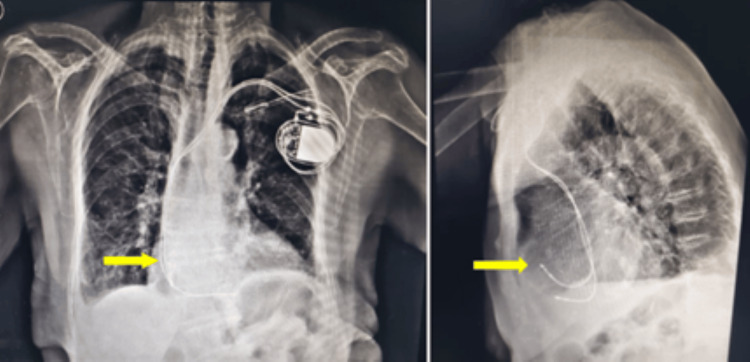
Posteroanterior and Lateral Chest Radiographs Chest radiographs (posteroanterior and lateral views) showing the pacemaker generator in the left subclavicular position with leads correctly positioned in the right heart chambers - the atrial lead anchored to the anterolateral wall of the right atrium and the ventricular lead directed toward the septal or apical region of the right ventricle. The cardiac silhouette appears mildly enlarged, with elongation and calcification of the aortic arch, increased bronchovascular markings, and a subtle basal interstitial pattern. No evidence of pneumothorax or generator displacement (arrows indicate the anchoring site of the right atrial lead).
